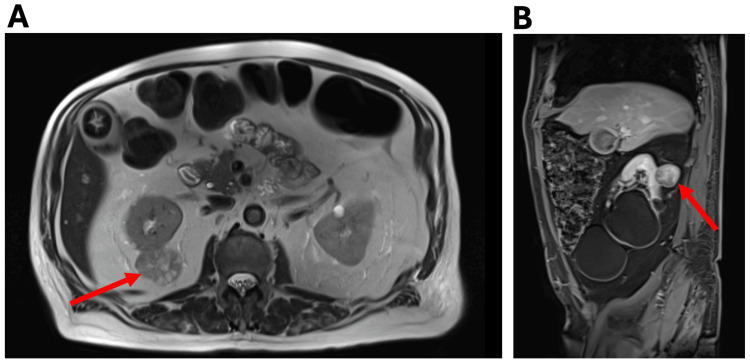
MRI of the abdomen (A and B) revealing an enhancing posterior upper-pole right renal mass measuring 4.1 × 3.6 × 3.1 cm, radiographically consistent with renal cell carcinoma. Image A is a transverse view and image B is a sagittal view. The red arrows indicate the location of the renal mass.
